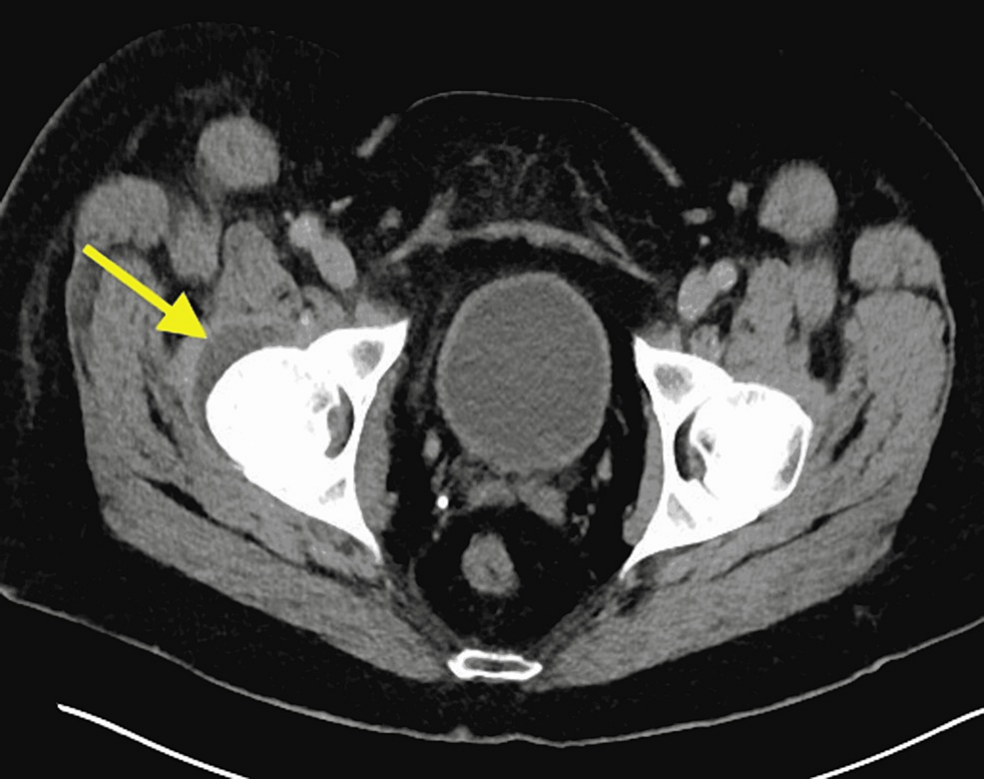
CT abdomen and pelvis with contrast demonstrated persistent right hip effusion.
